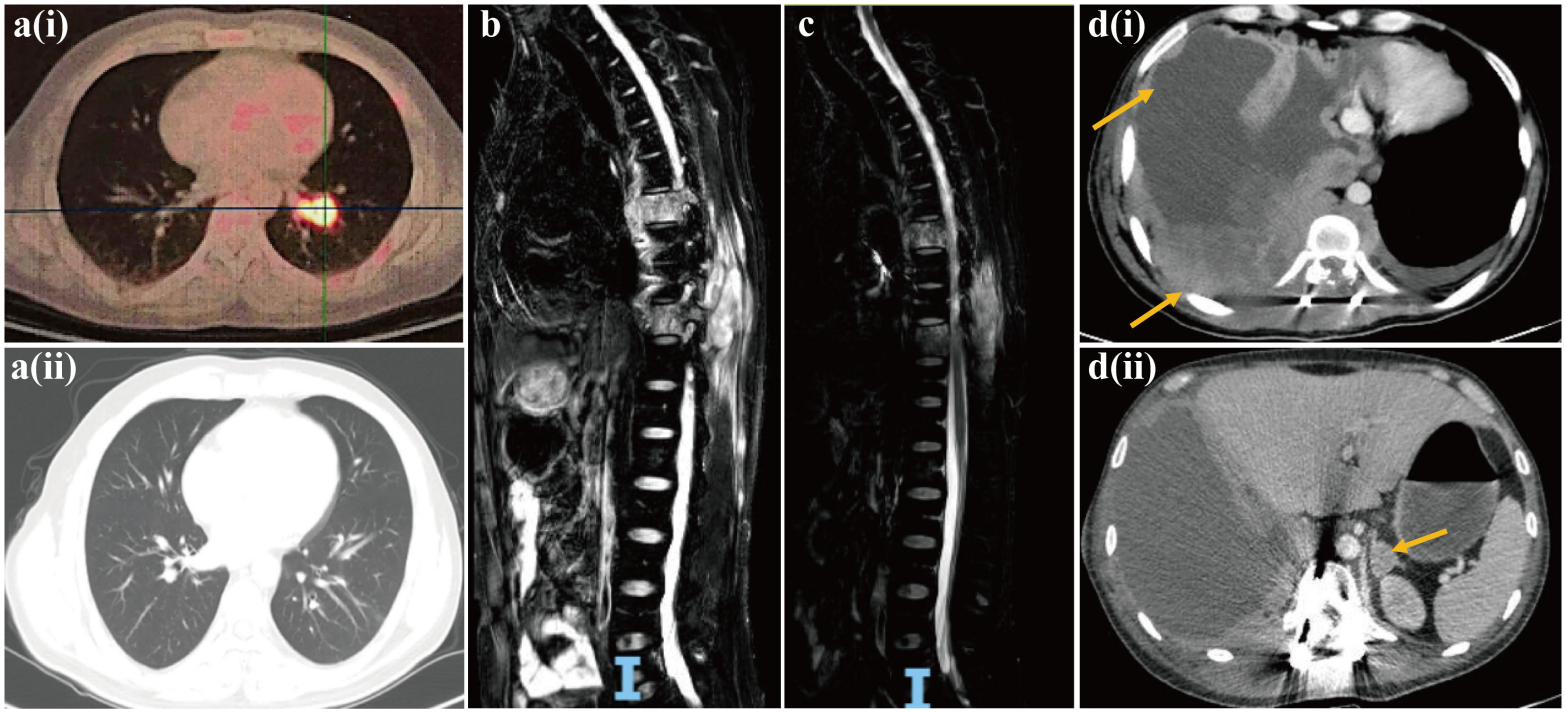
Computed tomography images of pulmonary NUT carcinoma pre-[a(i)] and post [a(ii)] two treatment cycles of chemotherapy (etoposide plus carboplatin) in combination of PD-1 inhibitor sintilimab. Magnetic resonance image of progressive disease with multiple bone and soft tissue metastatic lesions, and worsening of bone destruction after 4 months of treatment with BET inhibitor (b). Magnetic resonance image of reduction in multiple soft tissue lesions, and the improvement of spinal stenosis at the seventh and tenth thoracic vertebrae following chemotherapy in combination of anti-angiogenesis agent bevacizumab and radiotherapy (c). Computed tomography images of multiple lesions developing in the pleura, chest wall [d(i)] and adrenal gland [d(ii)].
